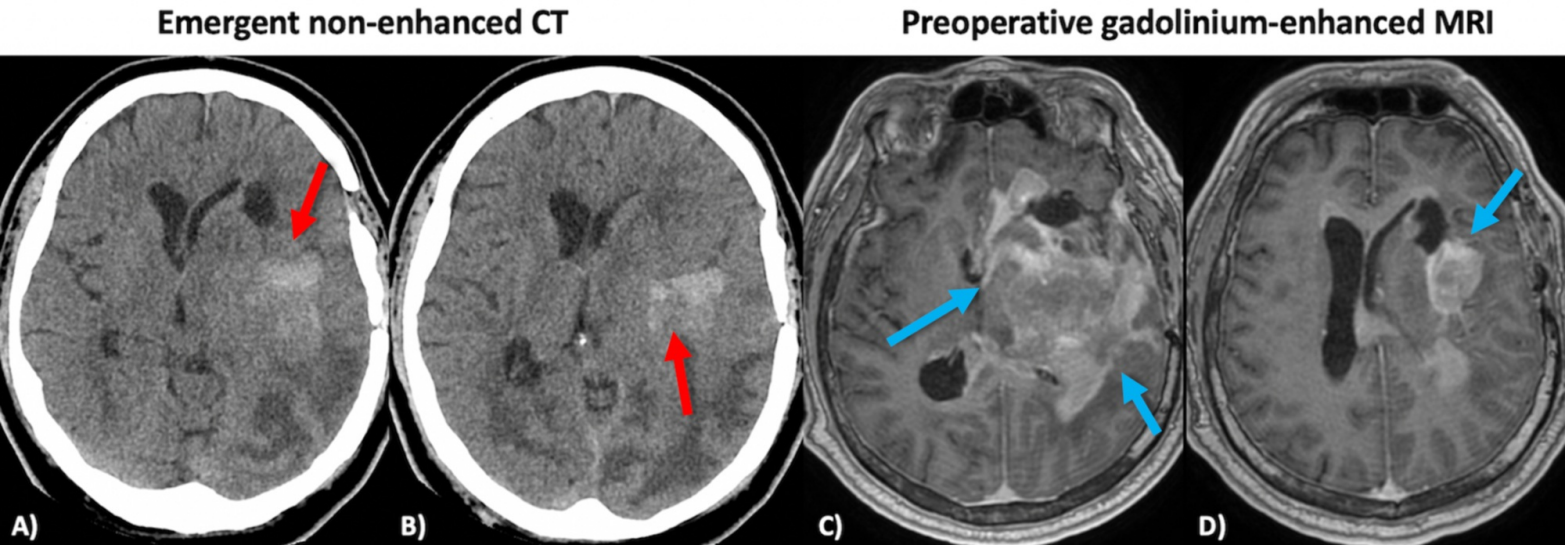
Emergent non-enhanced CT scan four years after the primary TBI (A,B) and gadolinium-enhanced MRI scan before stereotactic brain biopsy (C,D). Emergent non-enhanced CT revealed an atypically appearing spontaneous ICH in the area of traumatic scar, the same location where traumatic ICH has occurred back in 2014 (red arrows, slices A,B). Consequent gadolinium-enhanced MRI showed a heterogeneous, contrast-enhancing, and diffusely infiltrating mass (blue arrows, slices C,D). Glioblastoma, IDH negative was confirmed pathologically after the stereotactic brain biopsy. The GBM is centered in the same area where previous traumatic ICH occurred four years ago. ICH, intracerebral hemorrhage; IDH, isocitrate dehydrogenase; TBI, traumatic brain injury.
